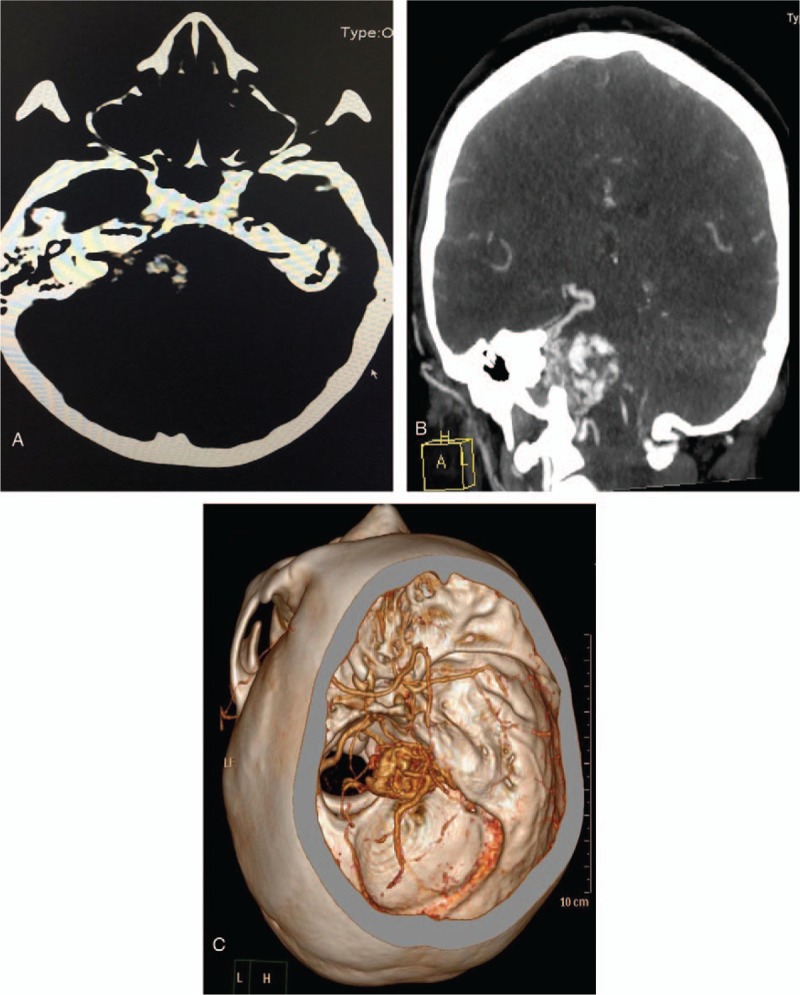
(A) A preoperative CT image showing expansion of the right auditory canal. (B and C) Preoperative CTA images showing the vasculature of the tumor. CT = computed tomography, CTA = computed tomography angiography.
